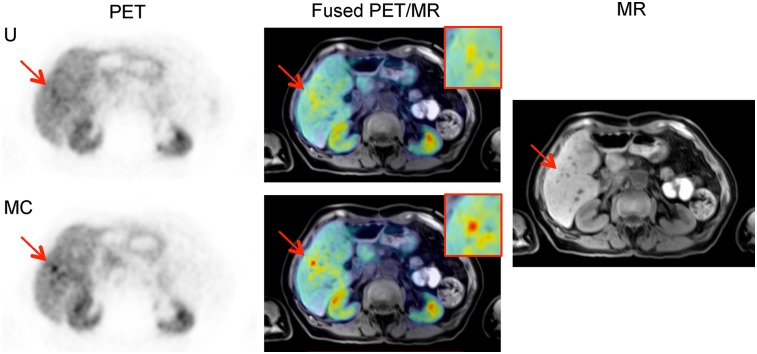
Case 1. Axial uncorrected (U) and motion-corrected (MC) PET images of lesion 1, along with T1-weighted Dixon volumetric interpolated breath-hold examination PET/MR image and MR image alone.
